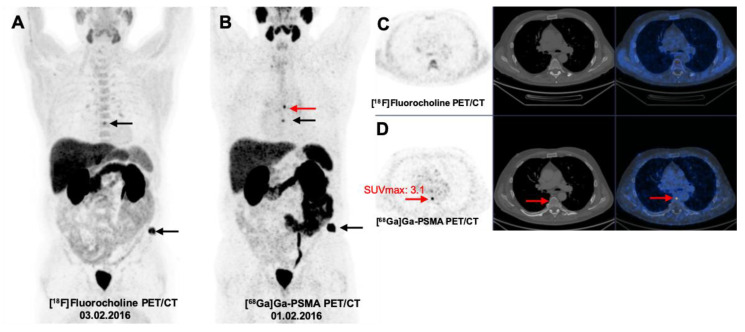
Prostate cancer recurrence: small skeletal metastases detected by [^68^Ga]Ga-PSMA PET/CT (Gleason score 8, PSA: 0.7 ng/mL). (A) [^18^F]Fluorocholine PET: maximum intensity projection (MIP) revealed multiple bone metastases (arrows) in the left ilium and T10 vertebra corresponding with the sclerotic changes on CT. (B) [^68^Ga]Ga-PSMA PET/CT: MIP detected additional small bone metastasis on T9 vertebra (red arrows) without relevant morphological changes on CT. (C) transaxial views: PET (left), CT (middle), and fusion PET/CT (right) images. (D) transaxial views: PET (left), CT (middle) and fusion PET/CT (right) images.
